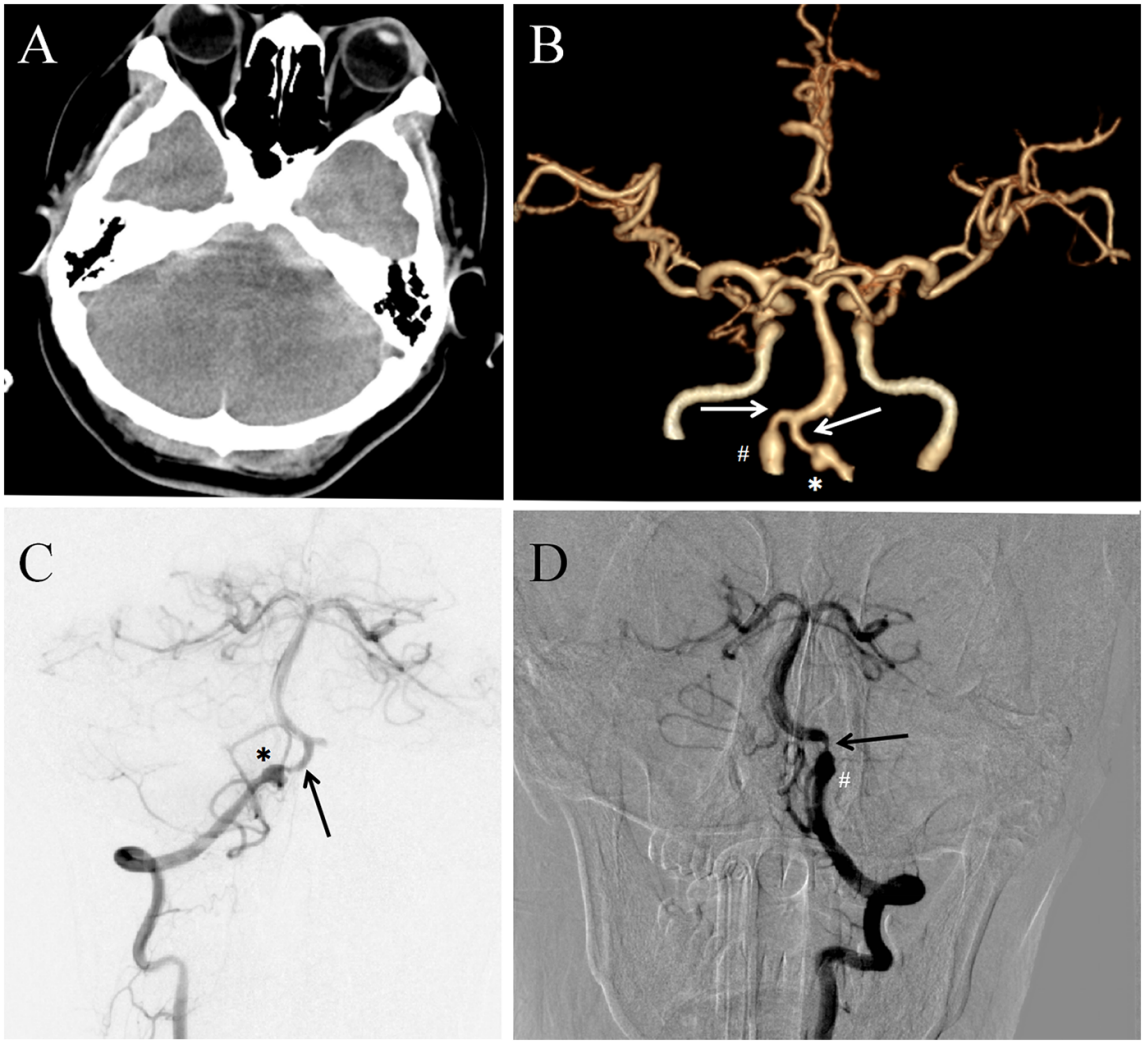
(A) Preoperative computed tomography of the head showed subarachnoid hemorrhage. (B) CT angiography (CTA) suggested a right ruptured vertebral artery dissection aneurysm marked by “*”. “#” indicated an unruptured dilated dissection of the left vertebral artery. Two arrows indicated severe stenosis at the ends of both vertebral arteries. (C) Digital subtraction angiography (DSA) identified a dissected aneurysm of the right vertebral artery with severe stenosis of the distal vessel. (D) DSA also showed an unruptured dissecting aneurysm and severe vascular stenosis in the left vertebral artery.
